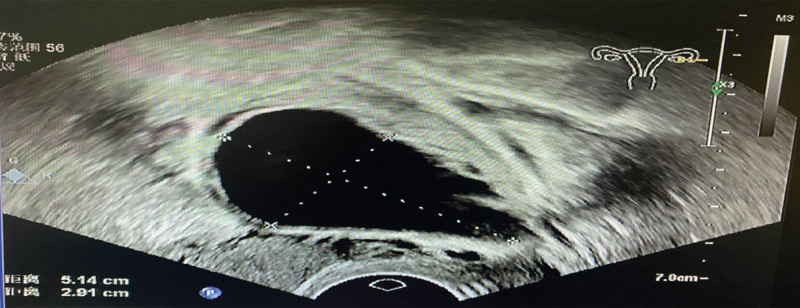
Transvaginal ultrasonography (case A) revealed a 5.1 × 2.9-cm cyst in the left ovary.
